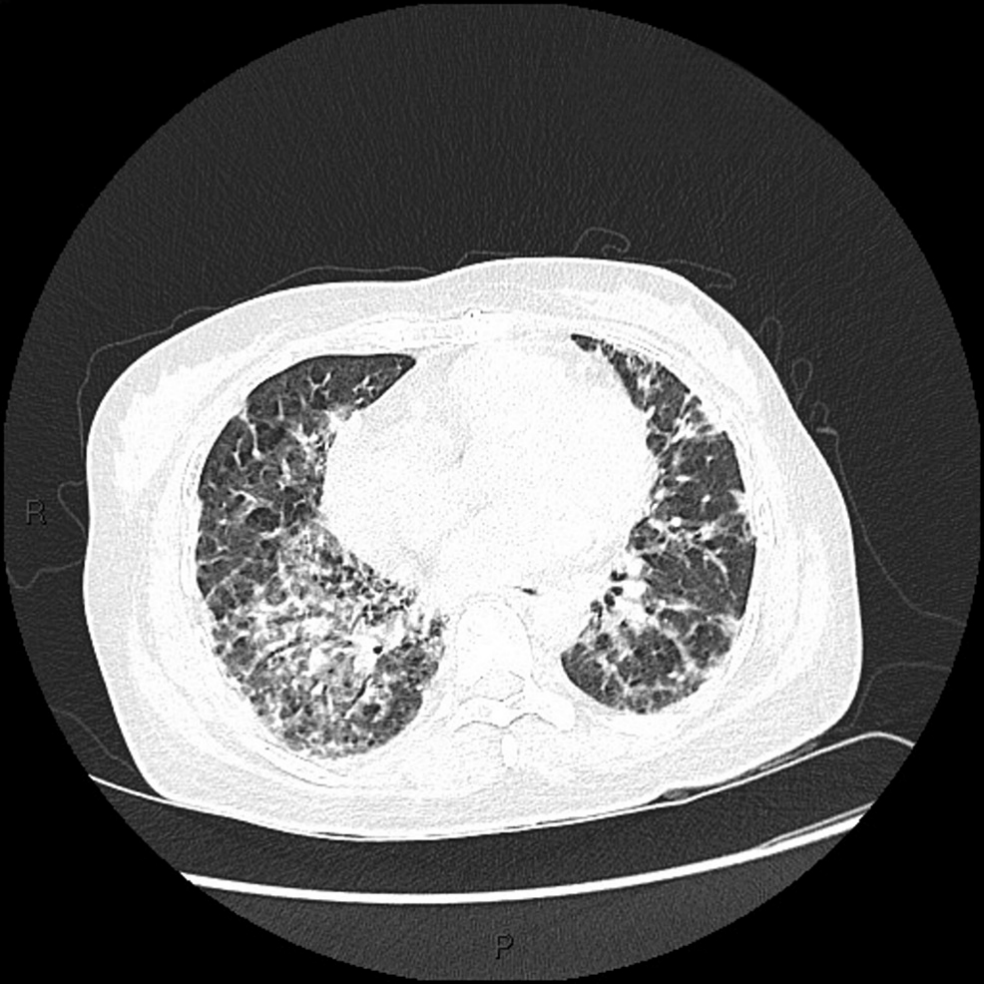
HRCT of patient 1 showing ground grass opacities in bilateral lung bases HRCT: High-resolution computed tomography.
